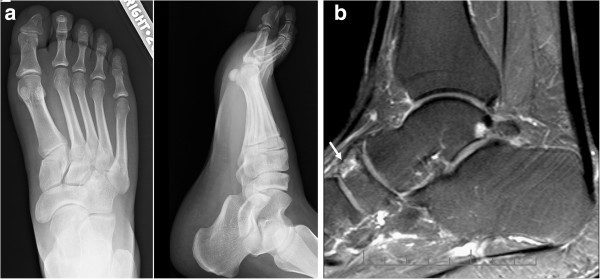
A 48-year-old patient with a history of asthma and hip osteonecrosis presented with a painful ankle. The plain x-rays (a, b) were negative. The sagittal fat suppressed T2-w TSE MR image (c) showed a small ON lesion in the navicular (arrow).
